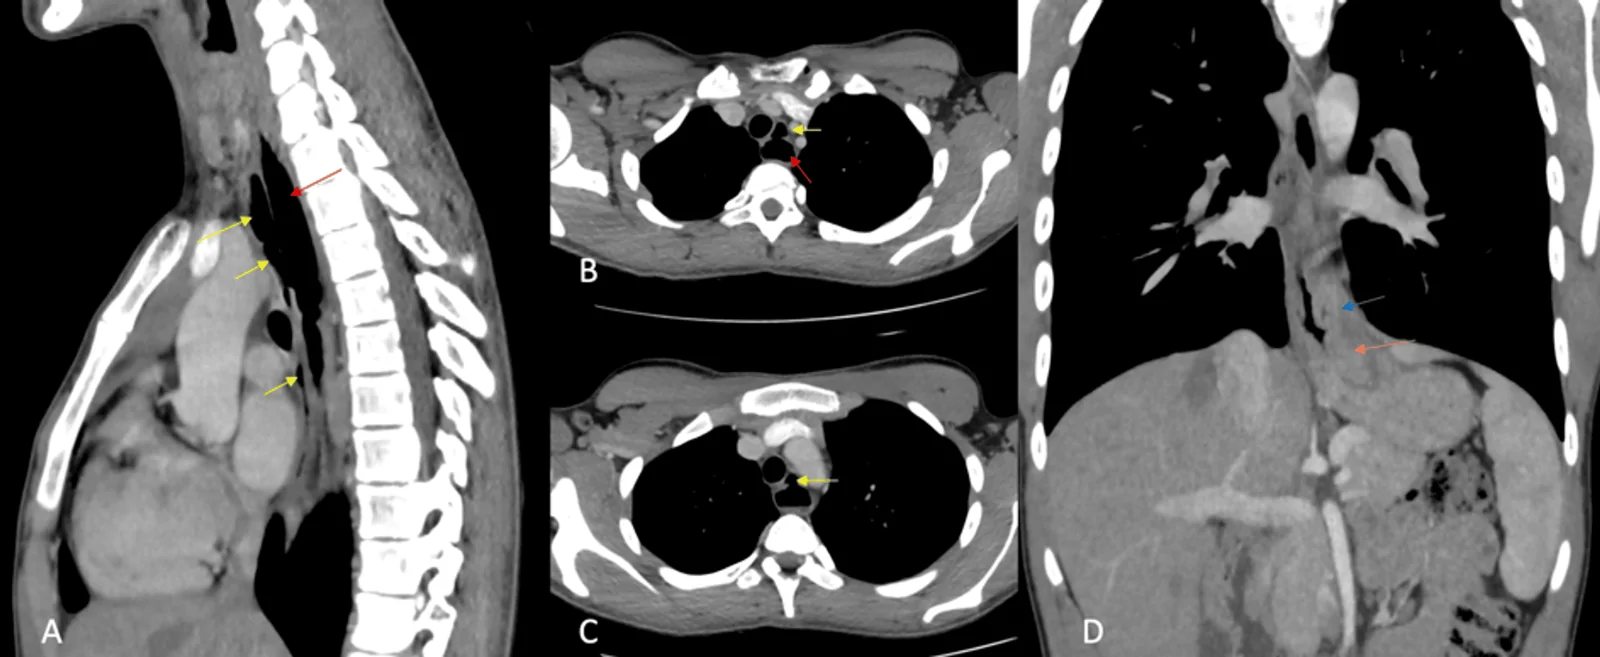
Contrast-enhanced CT acquired during the venous phase, with sagittal (A), axial (B, C), and coronal (D) reformations There is diffuse dilatation of the oesophagus, predominantly in the upper and middle thirds (red arrows), in the setting of a hiatal hernia (orange arrow). An air-filled structure lies anterior to the thoracic oesophagus, demonstrating three communications with the lumen in the upper third and two in the lower third (yellow arrows). This tubular structure shows mucosal contrast enhancement. There is mild, regular, presumed inflammatory thickening of the cardiofundic junction (10 mm) and the distal oesophageal wall (8.4 mm) (blue arrow). No abnormality of the mediastinal fat or cervical or thoracic lymphadenopathy is observed.
